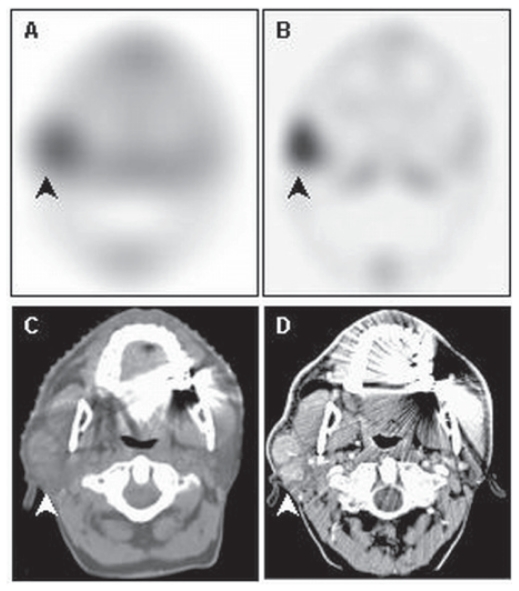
Baseline (A) and early post-radiotherapy (B) axial SPECT shows a prominent diffuse increase of tracer uptake in the right parotid gland. This change in uptake hampers the evaluation of tracer uptake in the low grade lymphoma of the left parotid gland as presented in the axial CT scan with (D) and without (C) contrast enhancement.
